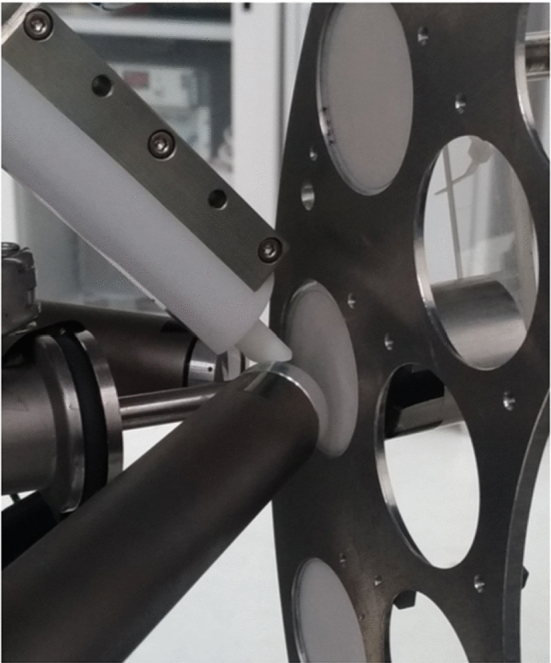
View of the set-up for external beam PIXE-PIGE measurements of atmospheric aerosol samples on the − 45° beamline at LABEC, with the SDD for low-energy X-ray detection (upper part of the picture, showing also the magnetic proton deflector assembly), the twin SDDs for mid- high energy X-ray detection (on the left and the right of the beam extraction nozzle), the filter holder wheel (with daily aerosol samples) and the Faraday cup behind it. The PIGE detector is not shown in this picture
